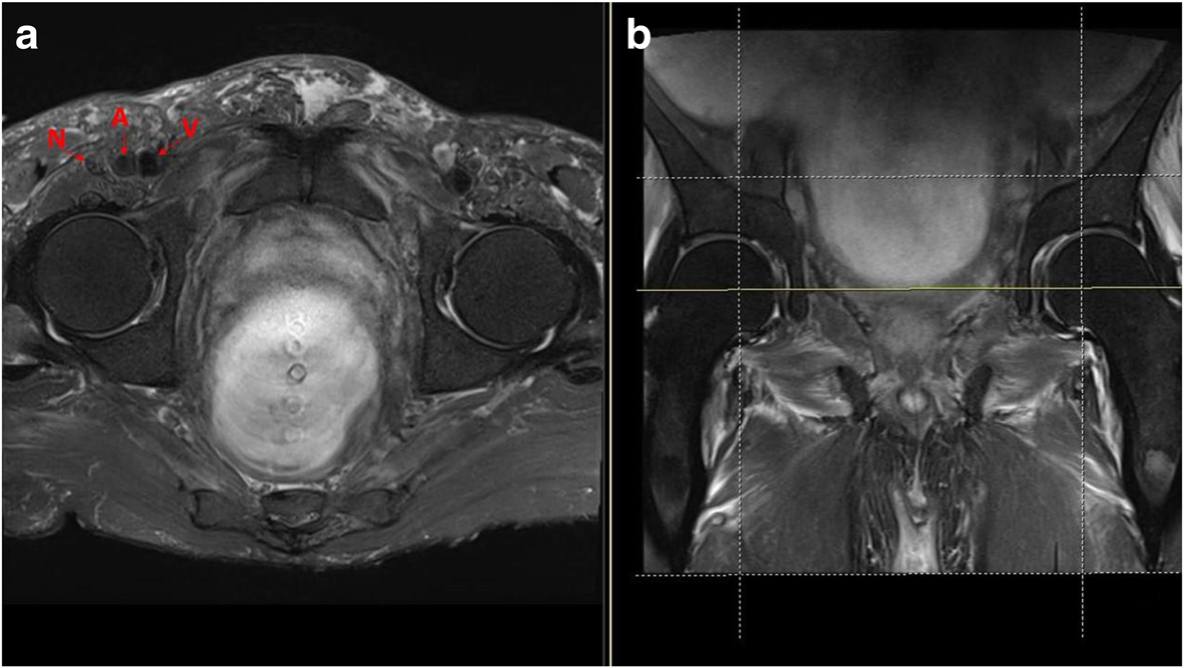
a Identification of structures on MRI. N femoral nerve, A femoral artery, V femoral vein. b Yellow line indicates the level of the axial slice used
